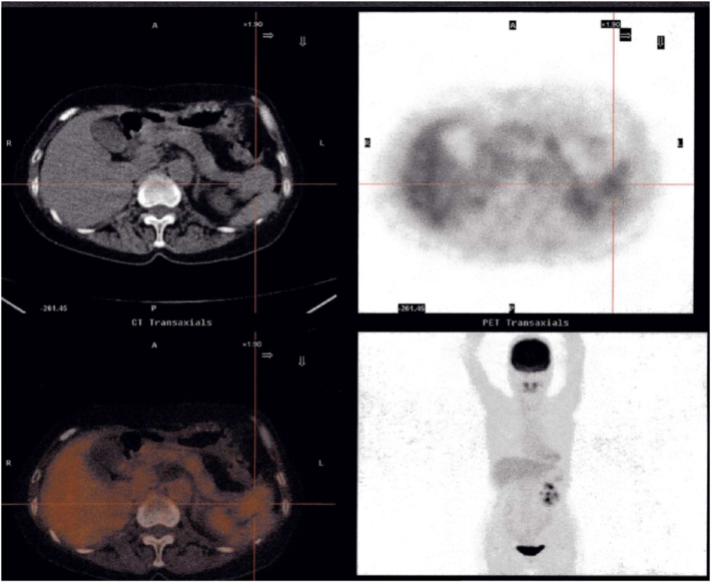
^18^F-2-fluoro-2-deoxyglucose positron emission tomography/computed tomography showing ^18^F-2-fluoro-2-deoxyglucose accumulation in the tail of the pancreas (standardized uptake value, 2.5)
